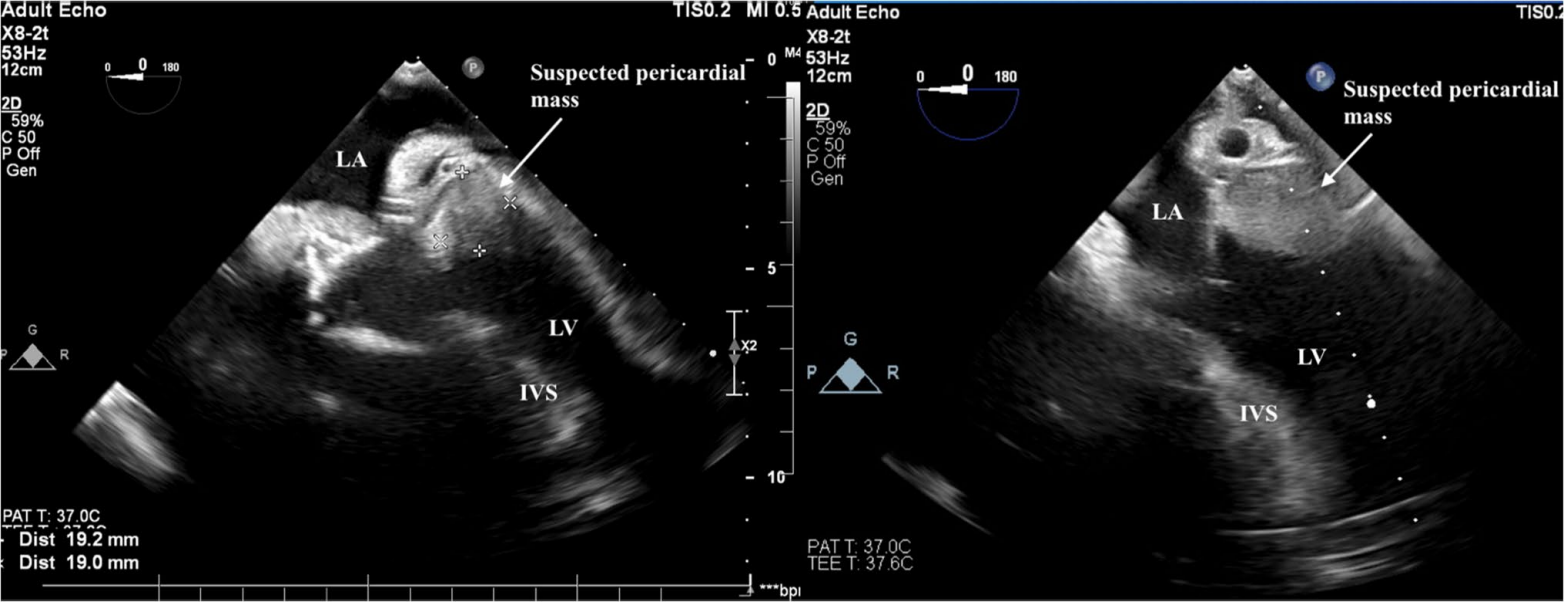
Echocardiography showing external compression to the left ventricle. LA, left atrium; LV, left ventricle; IVS, interventricular septum
